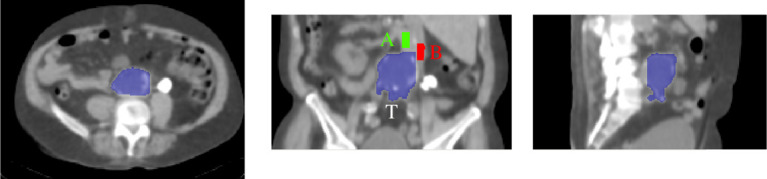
Extracted regions of interest (ROIs) showed on the CT image: the target AAA region (T), non-AAA aorta (A) and blood pool region or vena cava (B). (Online version in colour.)
